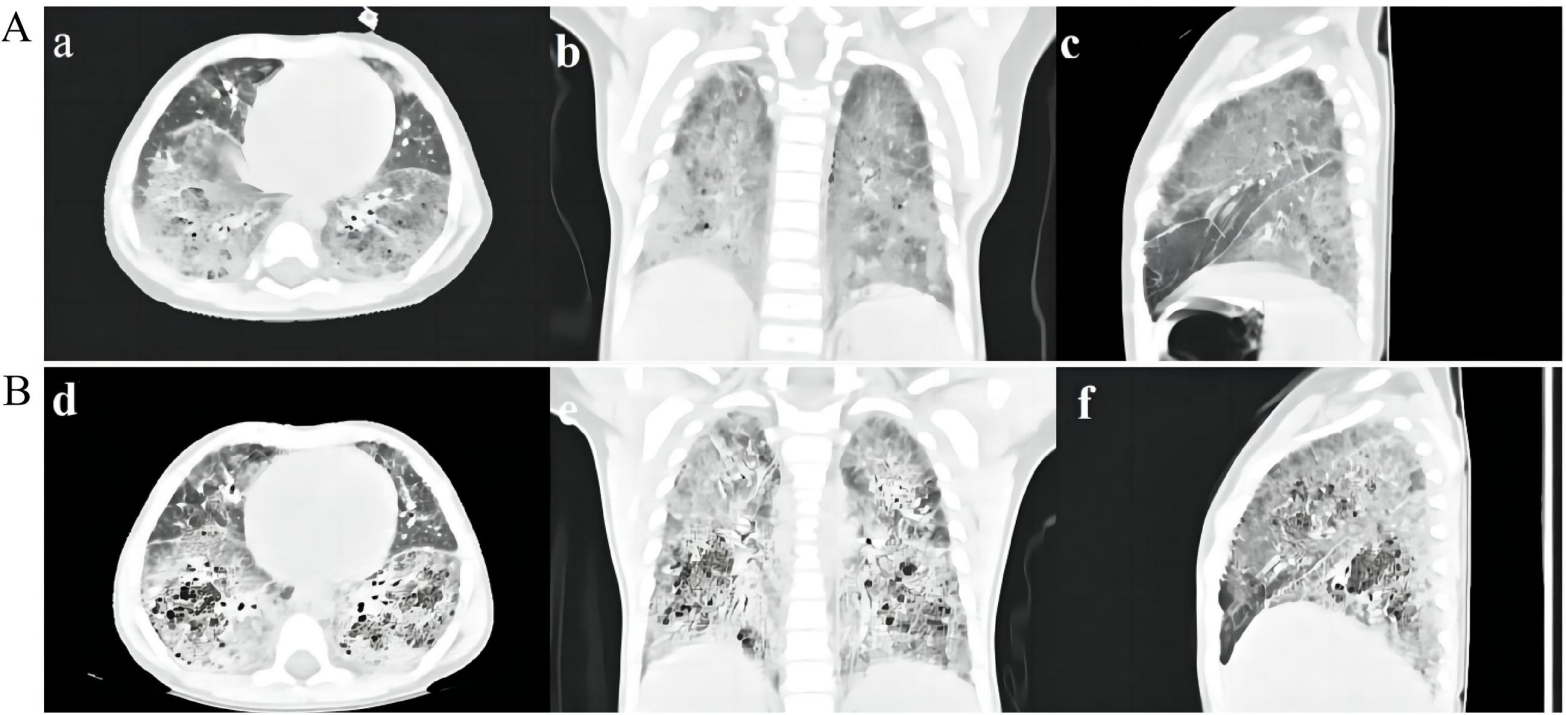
(A) HRCT scan of the patient. HRCT scan at 7 months of age showing diffuse reticular and bilateral asymmetrical ground-glass opacities (a–c). (B) HRCT scan 2 months after discharge showing multiple cysts and crazy-paving stone signs in both lung fields (d–f).
